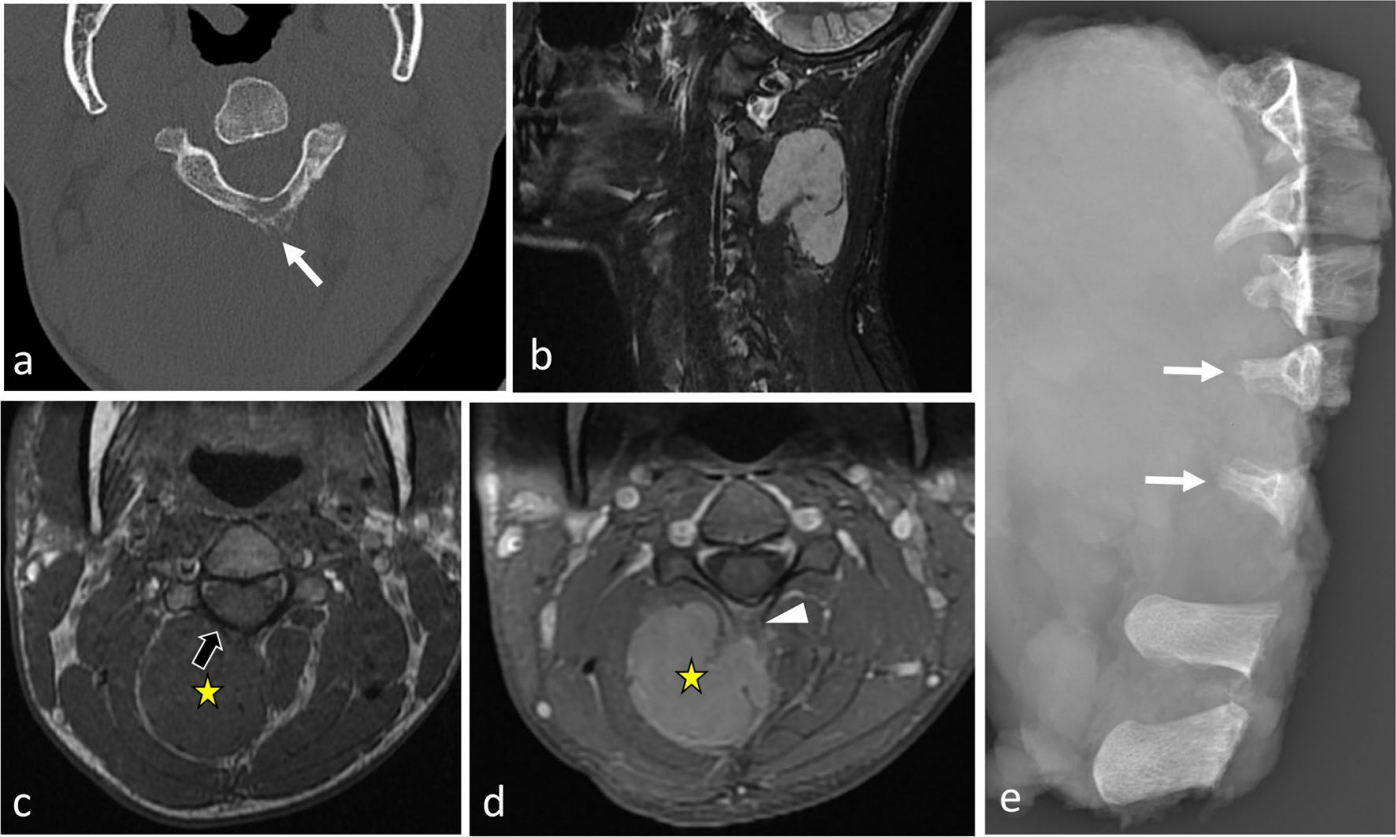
Synovial sarcoma* of deep epaxial muscles at C4 level in a 53-year-old male with a bump and acute non-mechanical cervical pain. The mass presents aggressive radiological features such as cortical and trabecular lysis of the neural arch of C4 (a) with moth-eaten margin. The mass has low intensity on T1WI (b), with enhancement on contrast-enhanced fat-suppressed T1WI (c) and high signal on T2WI (d). MRI features suggestive of bone invasion were: the disappearance of the fat plane separating the mass and the lamina (black arrow, c) the enhancement of medullary bone of the spinous process (white arrowhead, d) and the enhancement of periosteum of right lamina (black arrowhead, d). Lateral radiograph of the surgical sample (two-third vertebrectomy) (e) shows bone lysis of the tip of the vertebral processes (C4, C5 and C6) (white arrows). The yellow star shows the epicentre of the mass.
